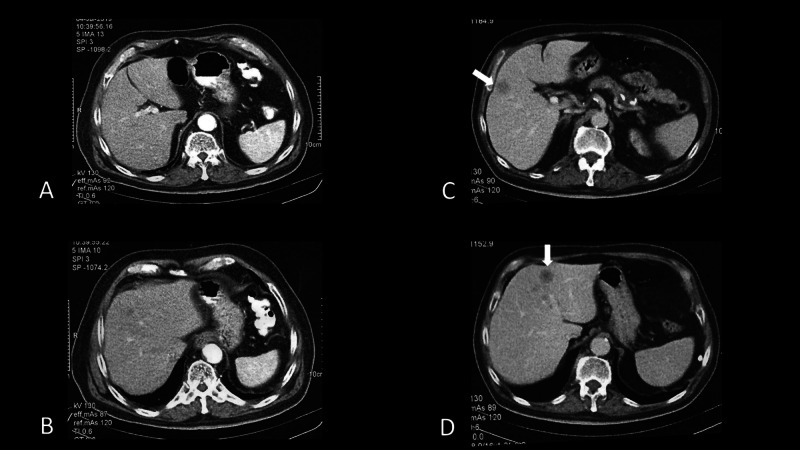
Liver metastases evident in the abdominal CT-scan examination at the time of documentation of sternal mass progression (C, D), a finding that was undetectable at CT-scans before the onset of immunotherapy (A, B).
